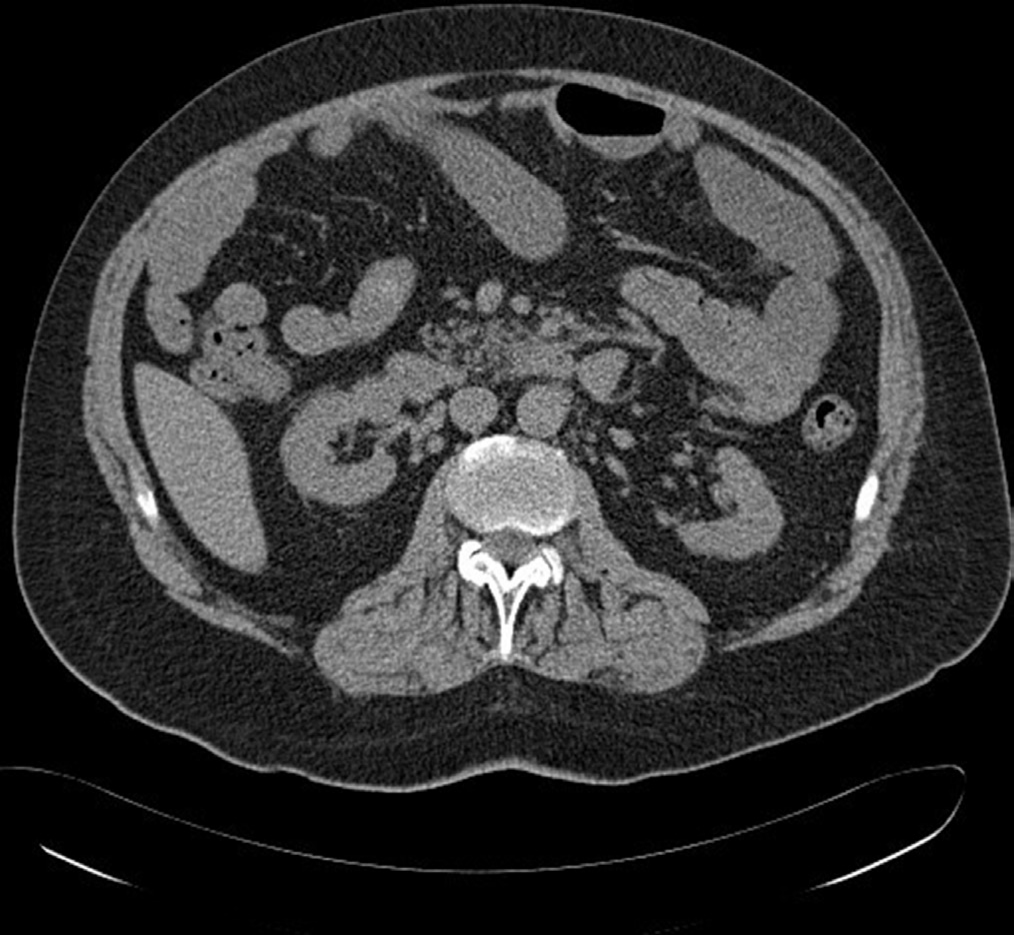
Axial view from a CT scan performed in 2008 showing the right kidney at the same level as the left kidney.
